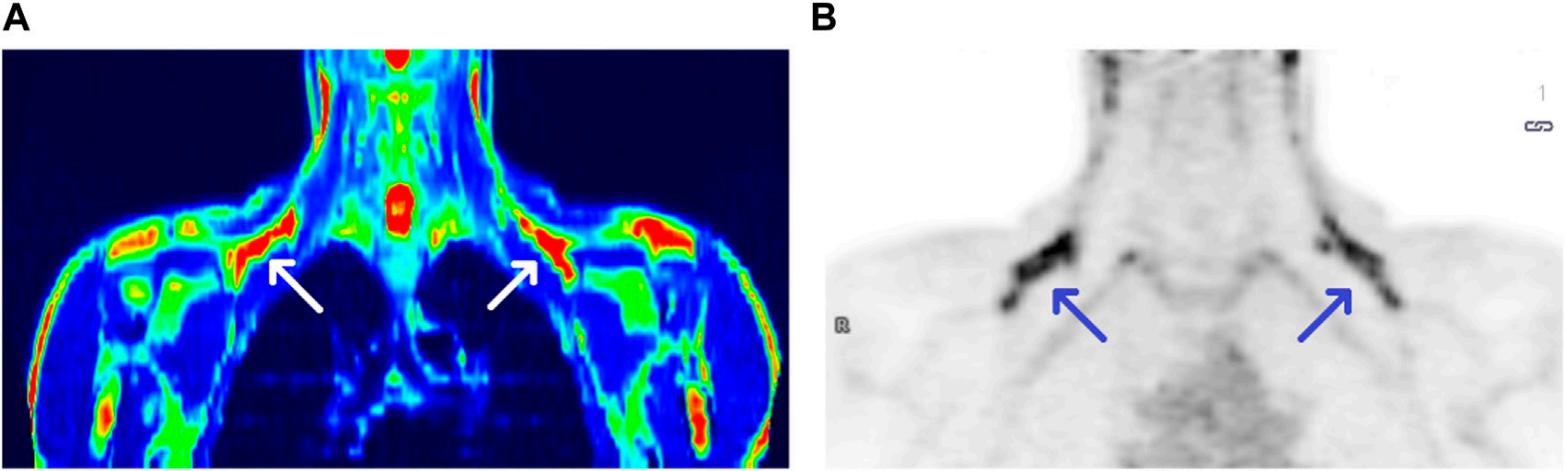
PET/MRI and PET scans of a BAT (+) individual [panel (A,B), respectively]. Arrows show BAT areas in supraclavicular regions.
